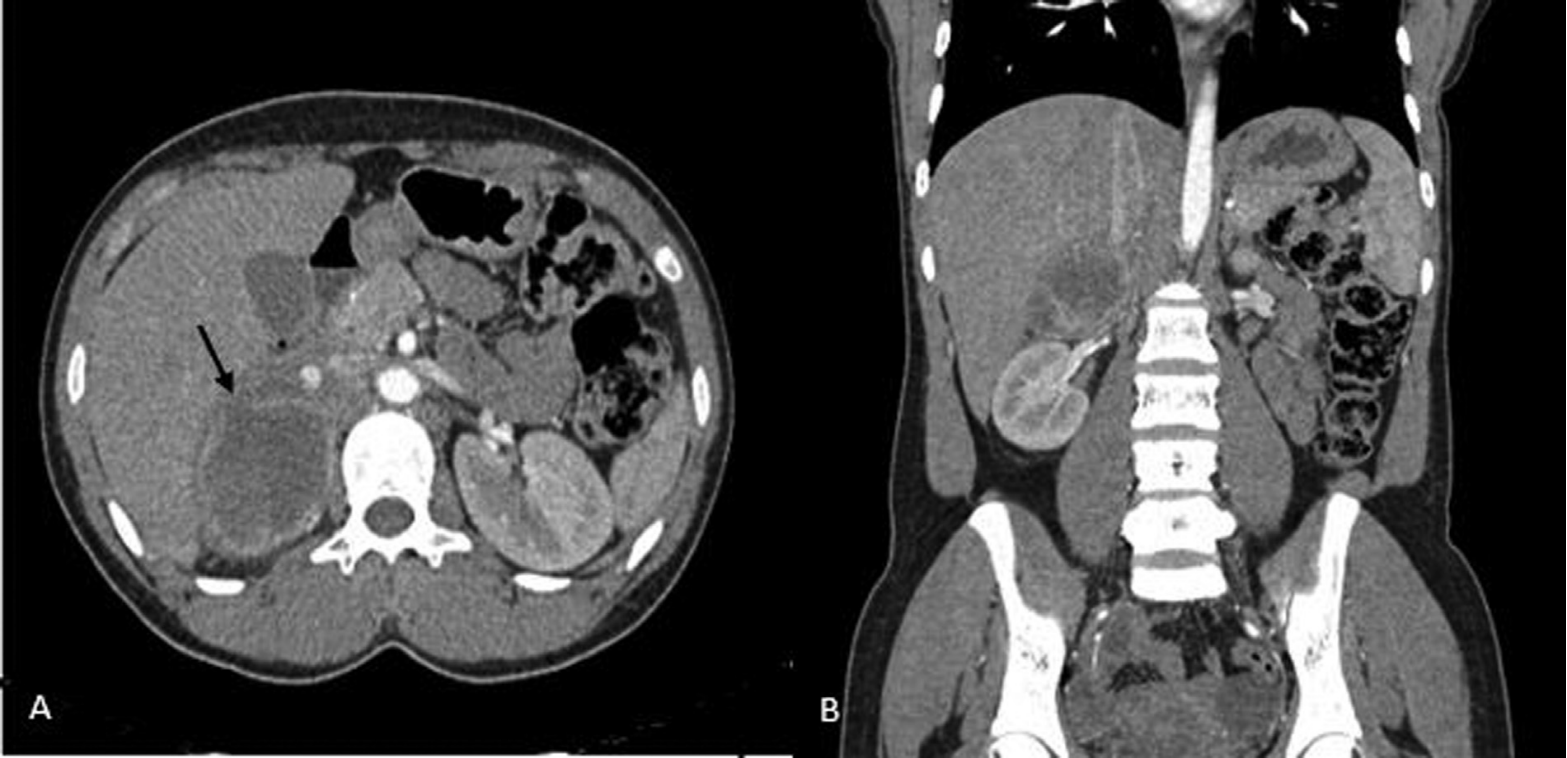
(A) Axial view and (B) coronal view of contrast-enhanced computed tomography showing a mass arising from the lateral limb of the right adrenal gland showing central liquefaction and intralesional hemorrhage with rupture (arrow) of the anterior wall leading to a walled off fluid collection extending into the anterior pararenal and subhepatic space.
